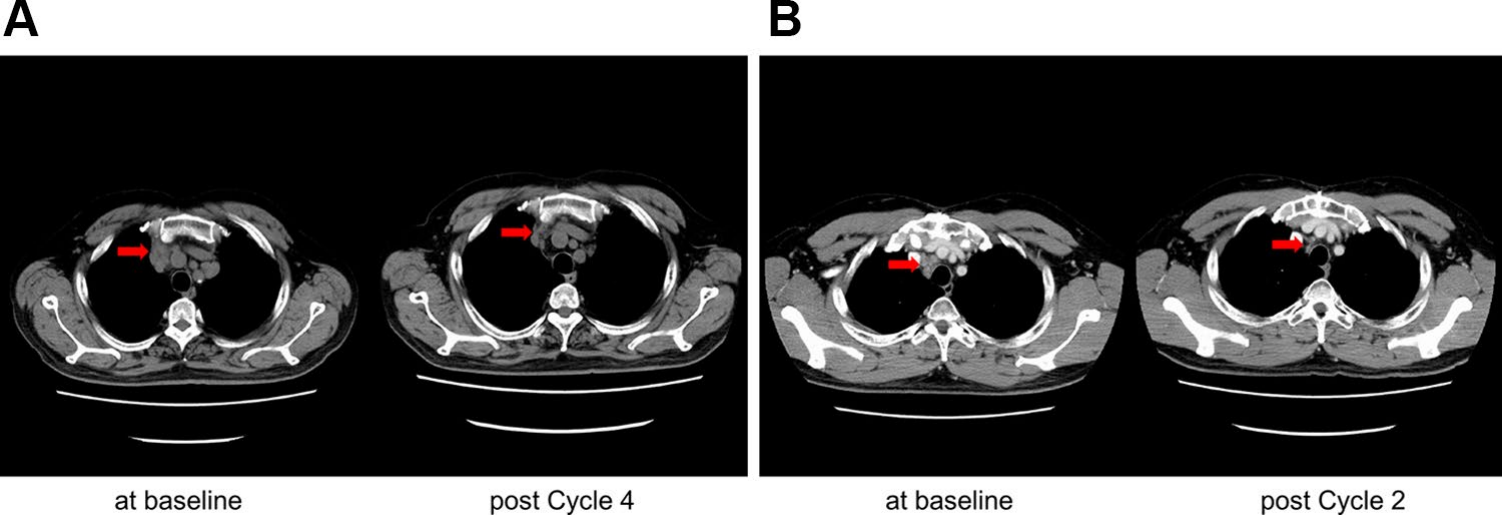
CT imaging in patients who experienced partial response. A S07 Prostatic small cell carcinoma treated with 75 mg/m^2^/day for 7 days and 14 days rest schedule; B S11 Thymic carcinoma treated with 75 mg/m^2^/day for 7 days and 14 days rest schedule; Tumor shrinkage was observed by a red arrow in both patients
